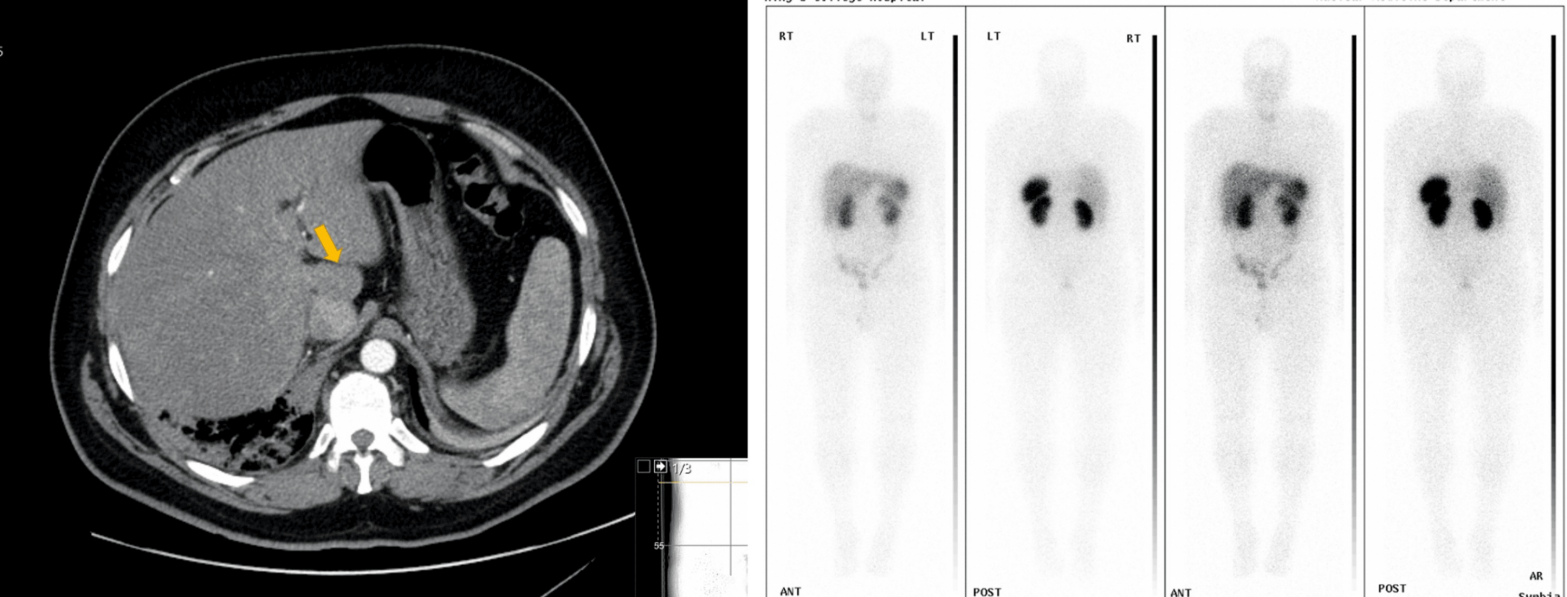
Abdominal mass on work-up, proven to represent UCD. On the left, CT scan showing a mass adjacent to the caudate lobe. On the right, MiBG scan showing no activity at the lesion. This case was treated in 2011, before the advent of DPET, which was introduced into clinical practice in 2016. MiBG: Meta-iodobenzyl-guanidine; DPET: DOTATATE positron emission tomography; UCD: Unicentric Castleman disease.
